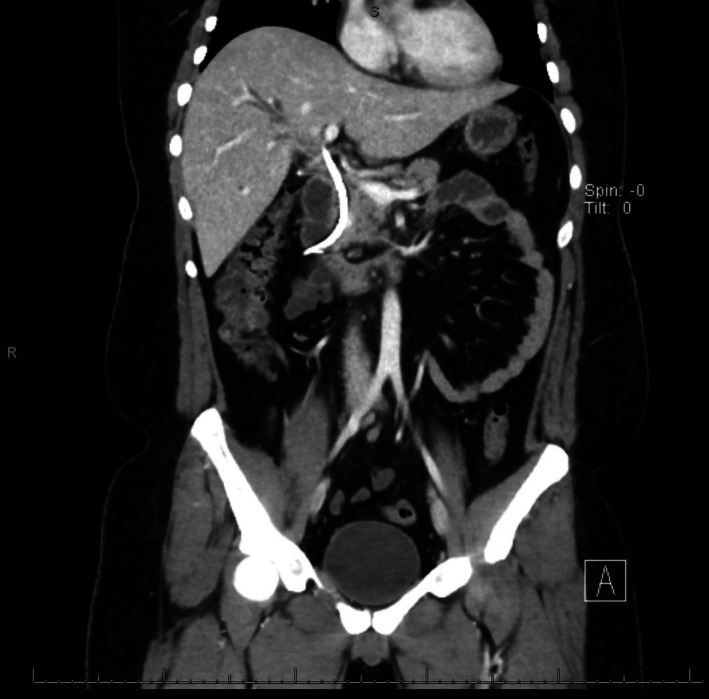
CT scan showing a CBD stent extending into the hepatic duct, with dilatation of the right hepatic duct and no visible dense stones (The image was edited by Paint 3D to hide the personal details of the patient).
